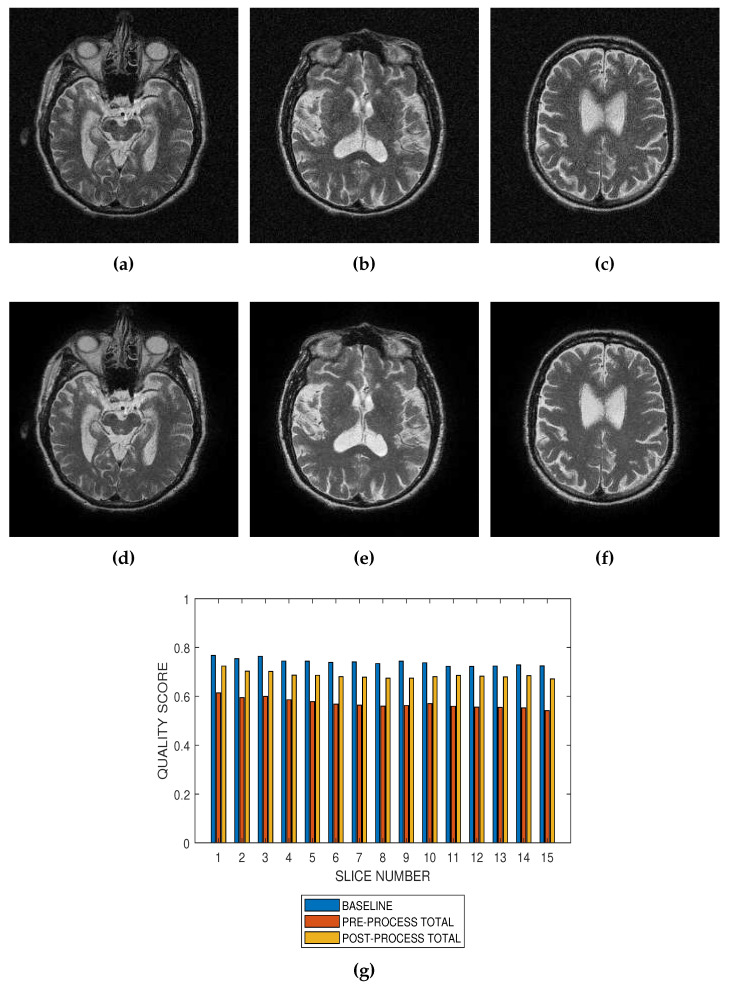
Application of the proposed method to the assessment of an image enhancement algorithm. (a–c) are slices numbers 20, 27 and 34, respectively in a T2 MRI volume degraded with 4 percent Rician noise. (d–f) are the slices displayed in (a–c) but their quality have been enhanced using a bilateral filter. (g) The baseline quality score of the original image, the predicted total quality indices of 12 successive T2 MRI slices in a volume data before and after enhancement with a bilateral filter.
